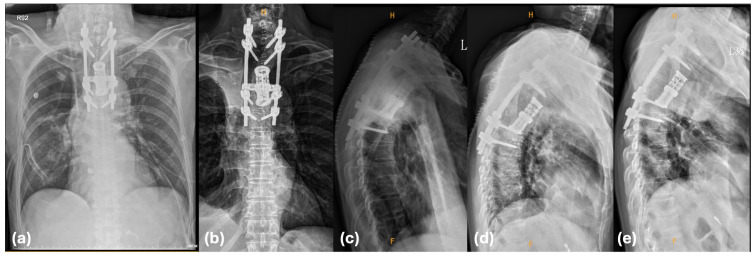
Postoperative radiographic surveillance. (a) Postoperative day 1 chest radiograph. (b) Anteroposterior (AP) radiograph at one month postoperatively demonstrating stable hardware positioning; Head (H); Foot (F). (c) Sagittal radiograph at one month, (d) two months, and (e) three months postoperatively confirming the maintenance of sagittal alignment and structural integrity of the expandable cage and posterior instrumentation.
